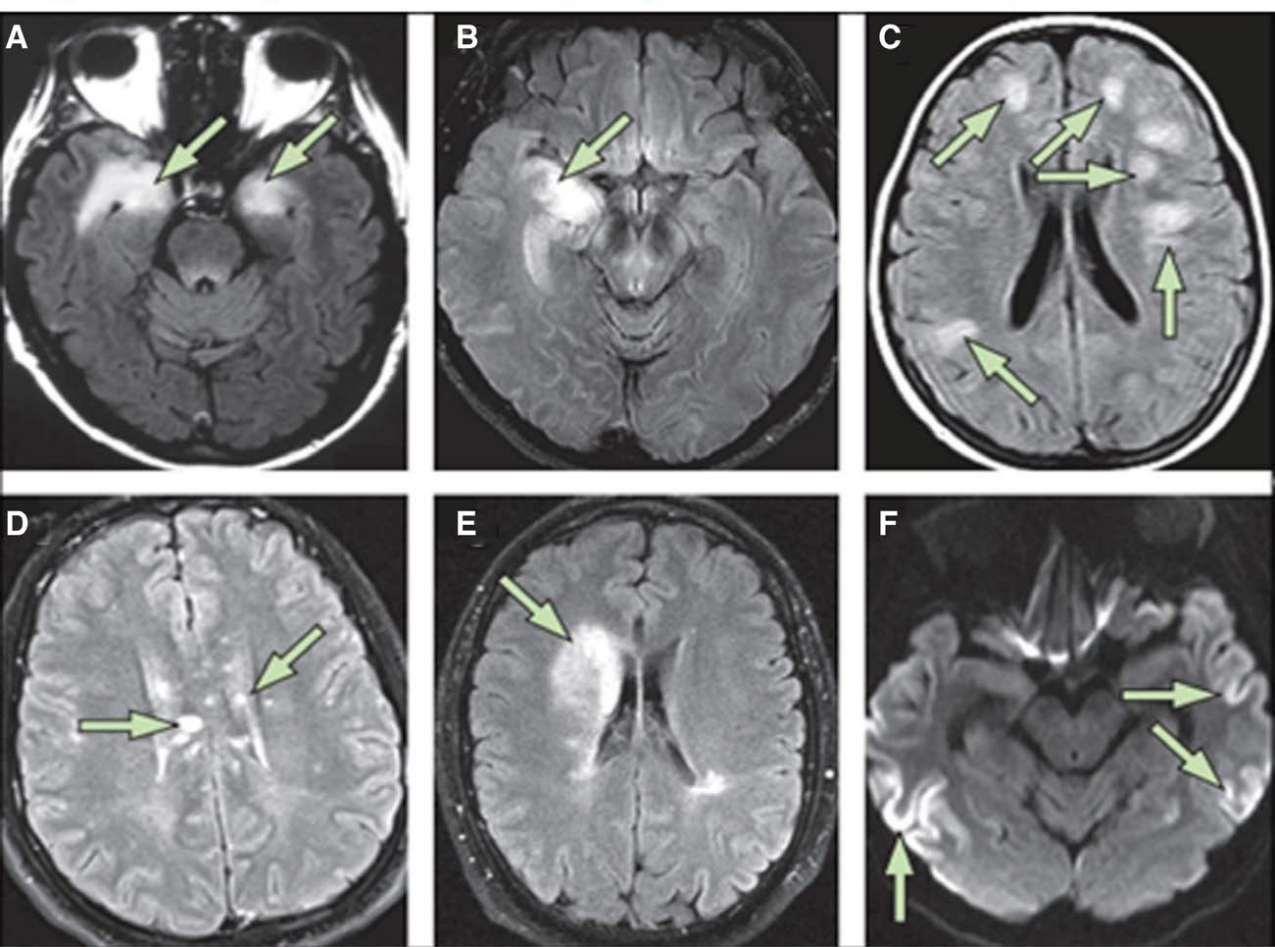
MRI sequences in autoimmune encephalitis and its mimics. Standard limbic encephalitis MRI (A) showing bilateral abnormalities in the medial temporal lobe on T2-weighted fluid-attenuated inversion recovery imaging; antineuronal antibodies were not detected in the serum or CSF of this autopsy-confirmed limbic encephalitis patient. The patient had a final diagnosis of glioblastoma (B) and showed signs of limbic encephalitis-like unilateral right hippocampus involvement. An acute disseminated encephalomyelitis (C) typical MRI shows bilateral massive lesions in the white matter. A patient suffering from Susac syndrome (D) has several lesions impacting the corpus callosum. An MRI of a patient with overlapping conditions (anti-NMDA receptor and myelin oligodendrocyte glycoprotein antibodies (E) reveals a demyelinating abnormality that is compatible with a right frontal. An MRI sequence that resembles the alterations observed in individuals with Creutzfeldt-Jakob disease is observed in a patient suffering from AMPA receptor antibody-associated encephalitis (F). AMPA = anti-alpha-amino 3-hydroxy 5-methyl 4-isoxazolepropionic acid, CSF = cerebrospinal fluid, MRI = magnetic resonance imaging, NMDA = N-methyl-D-aspartate.
